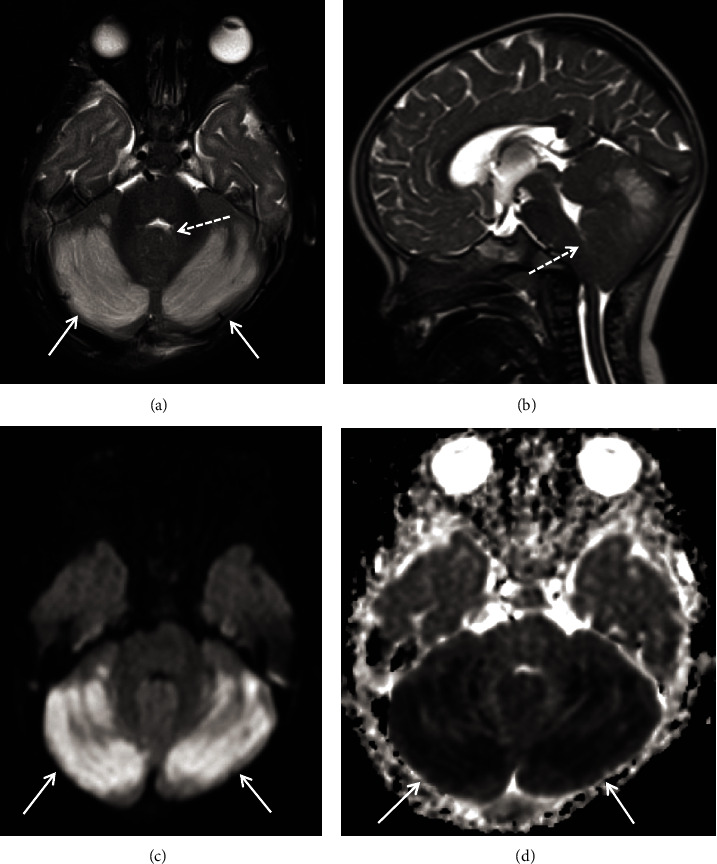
MRI at the time of presentation. (a) Axial T2-weighted image shows bilateral cerebellar edema (arrows) with effacement of the inferior fourth ventricle (dashed arrow). (b) Midsagittal T2 weighted image shows posterior fossa crowding with effacement of the inferior fourth ventricle (dashed arrow). (c) Diffusion imaging and (d) apparent diffusion coefficient (ADC) map show true restricted diffusion in the cerebellar hemispheres (arrows) in the region of T2 signal abnormality consistent with cytotoxic cerebellar injury.
